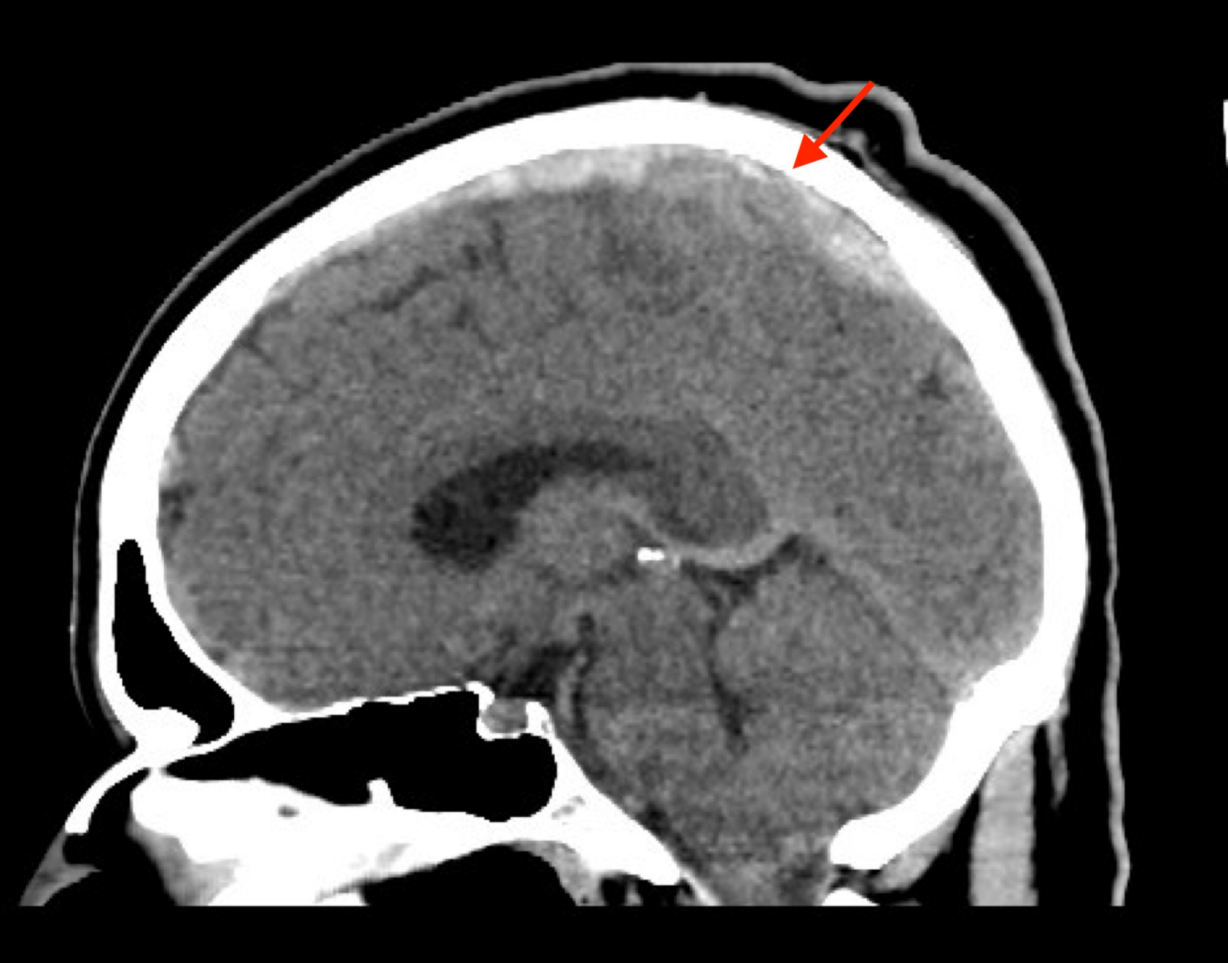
Sagittal non-contrast computed tomography (CT) of the thrombus demonstrating a cord sign
